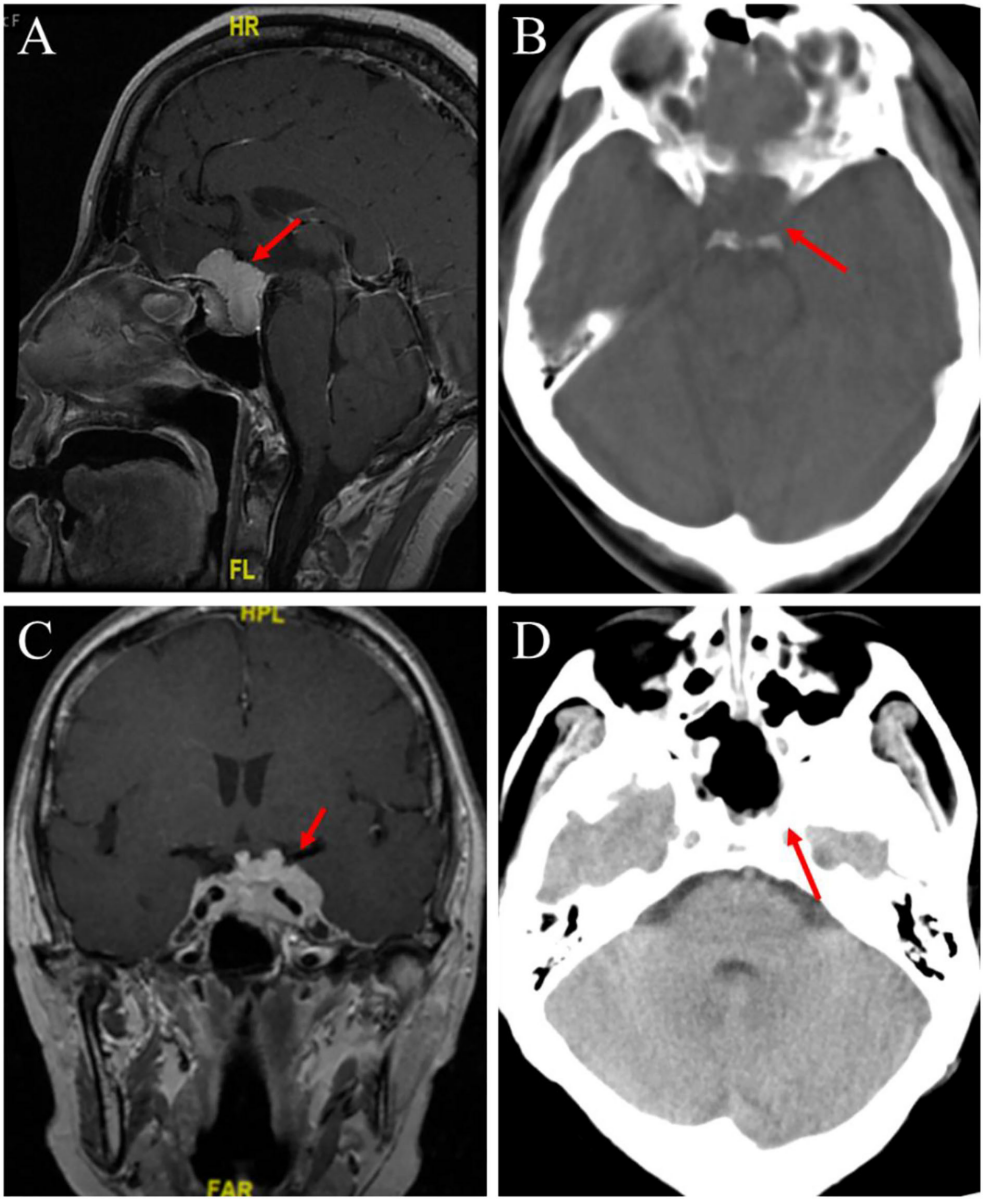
Preoperative head MRI and postoperative head CT examination were performed. (A) An enhanced MRI scan of the head before the first operation, the red arrow points to the pituitary fossa mass. (B) In the first postoperative CT scan, the red arrow points to the complete resection of the meningioma tumor. (C) T1-weighted enhanced coronal image before the second operation, the red arrow points to the same shadow of the newly formed T1T2 signal in the post-saddle region. (D) In the second postoperative CT scan, the red arrow points to the complete resection of the sellar mass.
